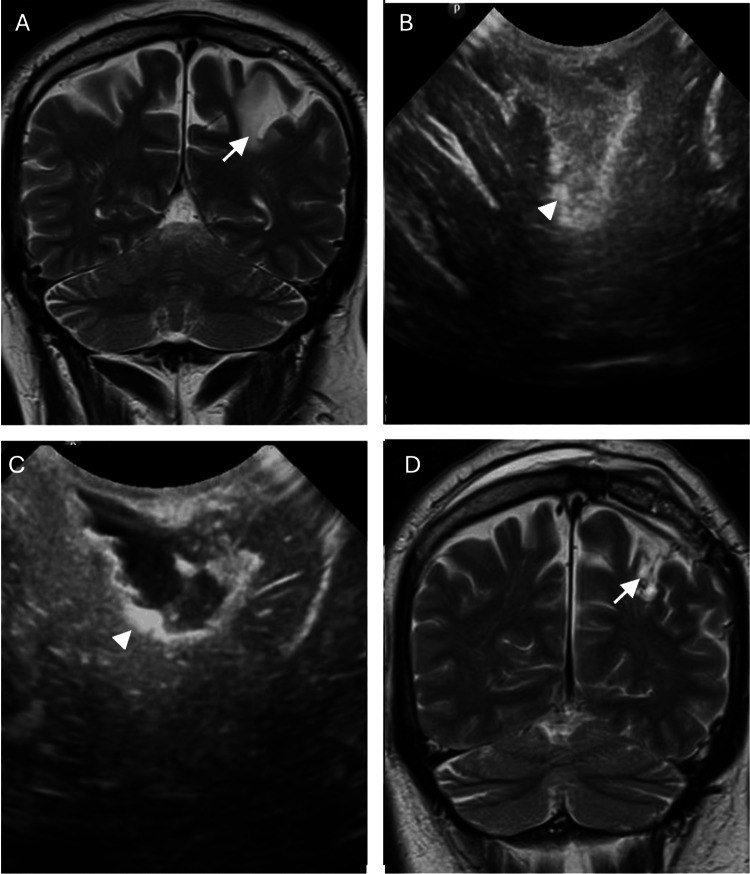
Left frontal low-grade glioma (A) Preoperative T2 MRI shows hyperintense intra-axial tumor (white arrow). (B) Intraoperative ultrasound shows hyperechoic intra-axial tumor with well-defined edges (white arrowhead). (C) Postoperative intraoperative ultrasound image shows total resection of tumor (white arrowhead). (D) Postoperative T2 MRI shows total resection of intra-axial tumor (white arrow).
